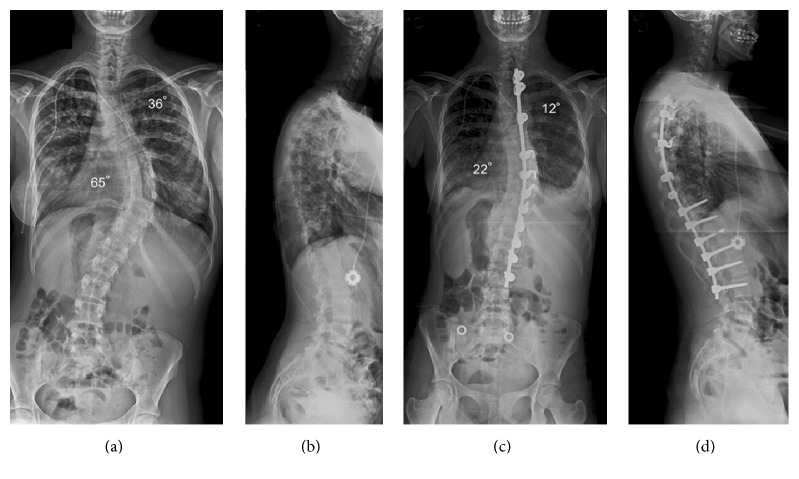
Preoperative posterior-anterior (a) and lateral (b) radiographs of the spine show a left upper and right low thoracic scoliosis measuring 36 and 65°, respectively. Posterior spinal fusion with the use of a right hook/screw and rod construct has achieved excellent correction of the curves to 12 and 22° and a satisfactory global coronal and sagittal balance for the spine (c, d).
